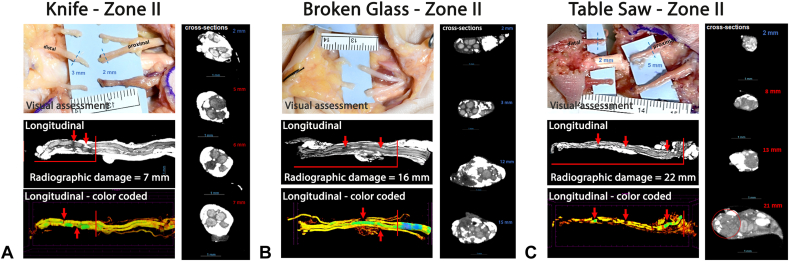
Representative images of A knife, B broken glass, and C table saw injuries. Images include visually estimated nerve damage (left top), original radiographic fascicular nerve damage measurement (left middle), color-coded radiographic fascicular nerve damage measurement (left bottom), and radiographic nerve cross-section for each laceration mechanism (right). Red arrows indicate areas of “skip” lesions.
